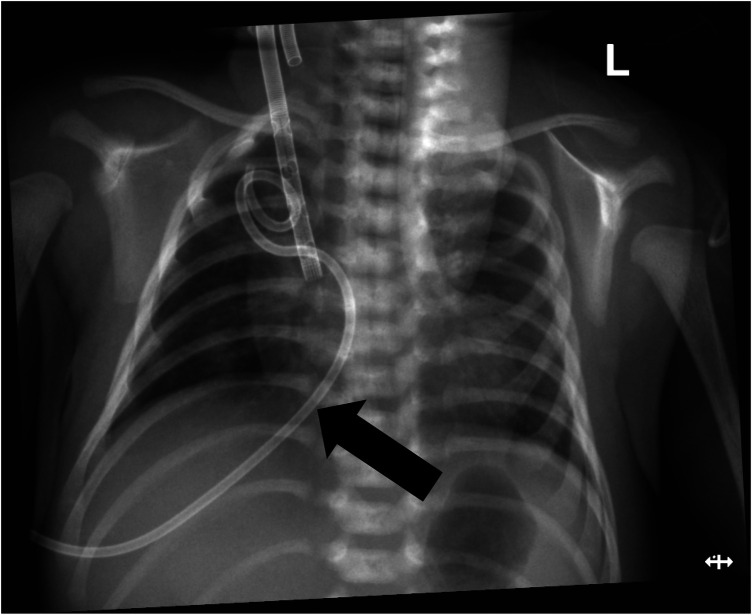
Chest x-ray showing resolved pneumothorax.
